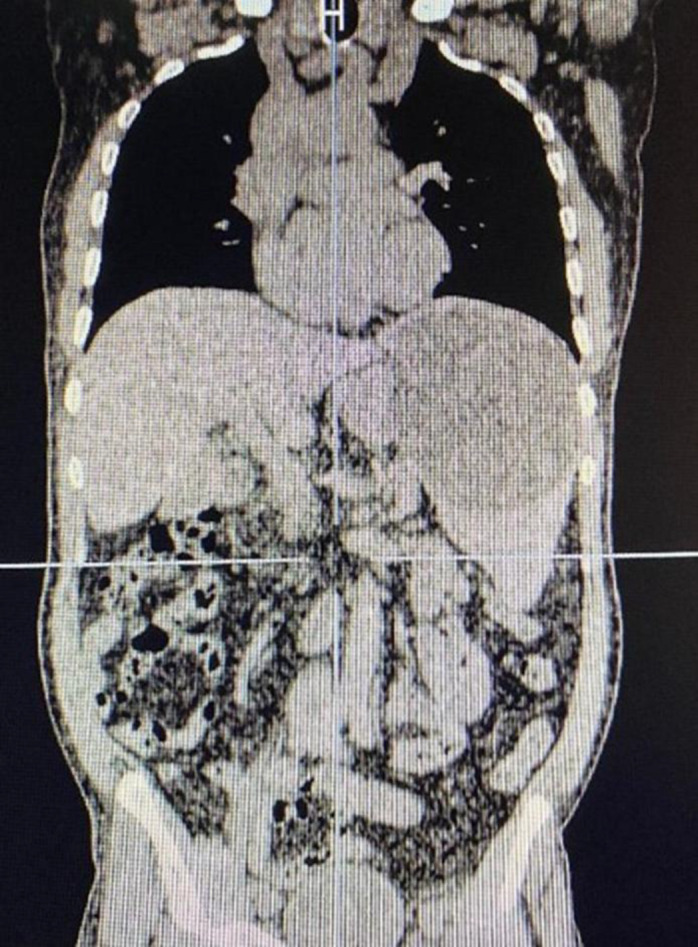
Based on the CT scan of the abdomen and pelvis, there were a large number of peritoneal nodules in different regions of the abdomen and in the middle part of the omentum and above the umbilicus, for which hydatid cysts, primary neoplastic or metastatic lesions to the peritoneum were included in the differential diagnosis.
